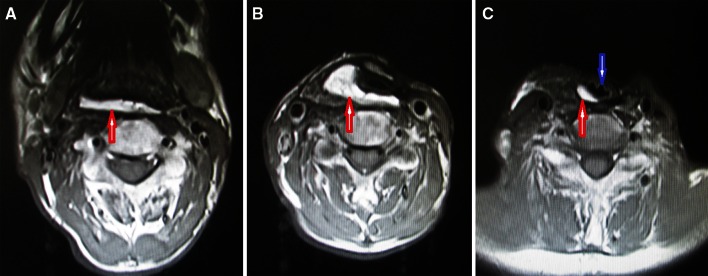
Results of postoperative MRI examination in the same patient following a total laryngopharyngoesophagectomy to remove a left piriform sinus lesion involving the cervical esophagus and reconstruction with gastric pull-up and pectoralis major flaps
